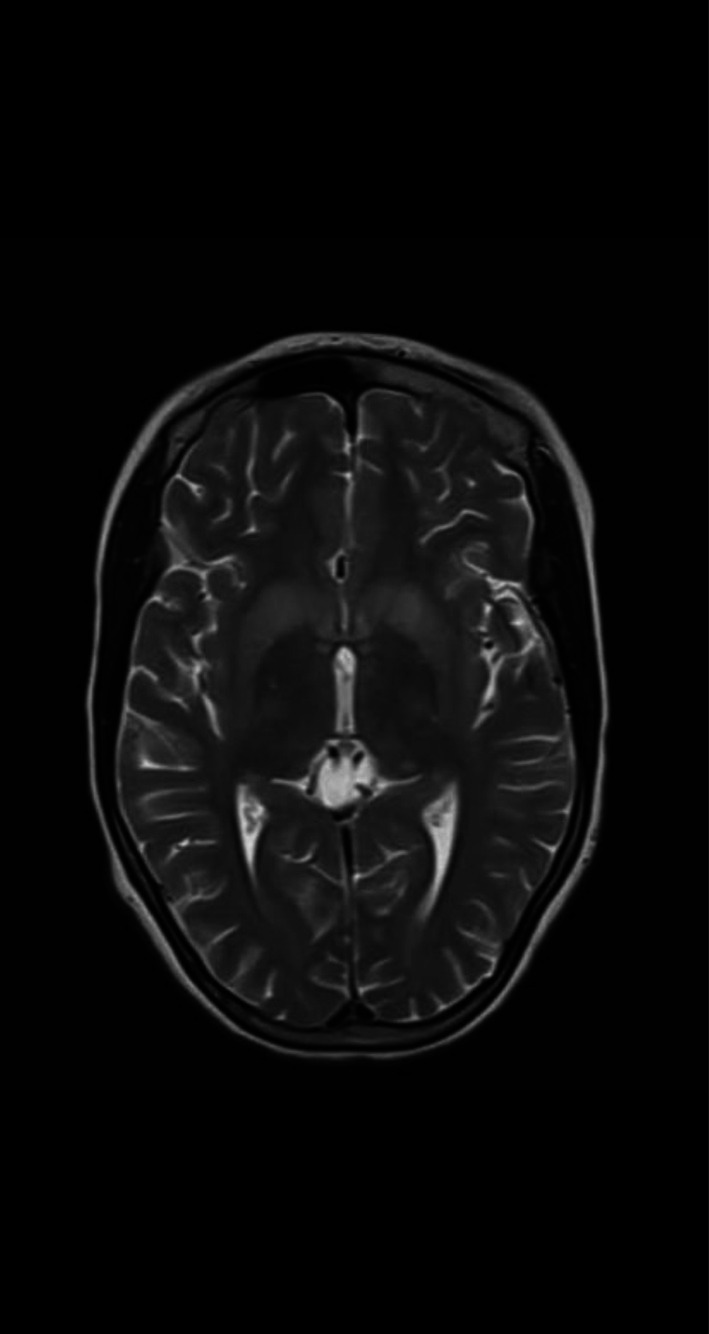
Axial T2 weighted MR image shows hyperintensity in the putamina, caudate nuclei, and ventrolateral thalami (which is the most common abnormality).
